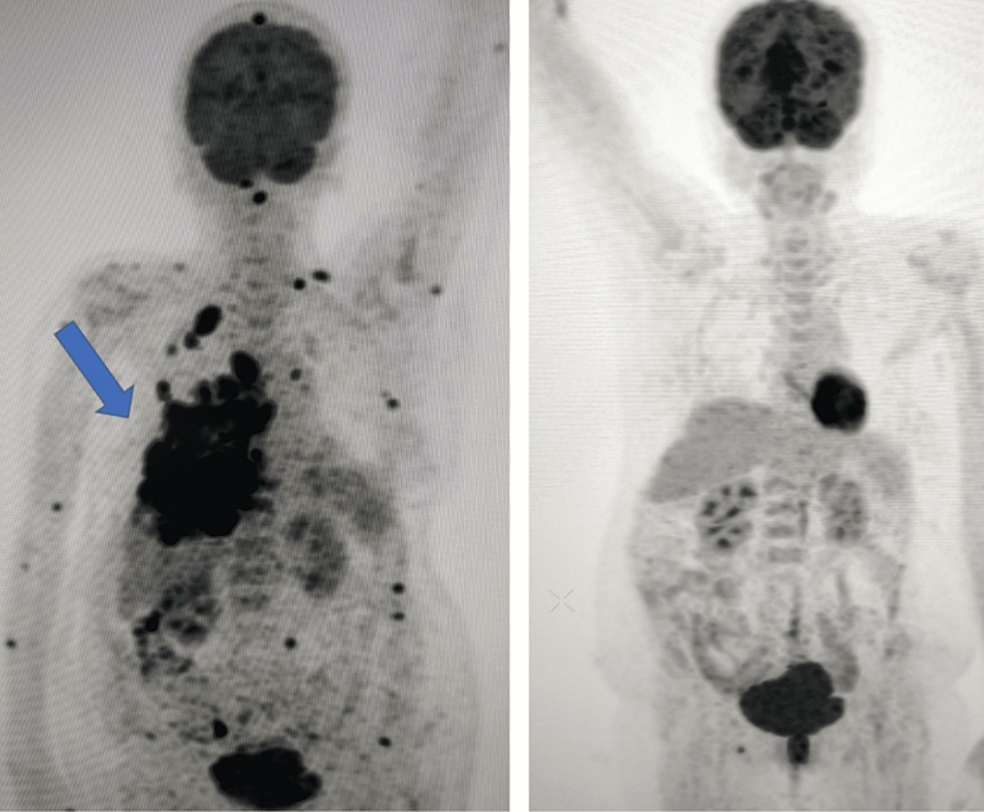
A comparison of baseline PET-CT scan (multiple skin and muscular lesions) with that after eight cycles of systemic therapy (complete metabolic response). PET-CT: positron emission tomography-computed tomography
